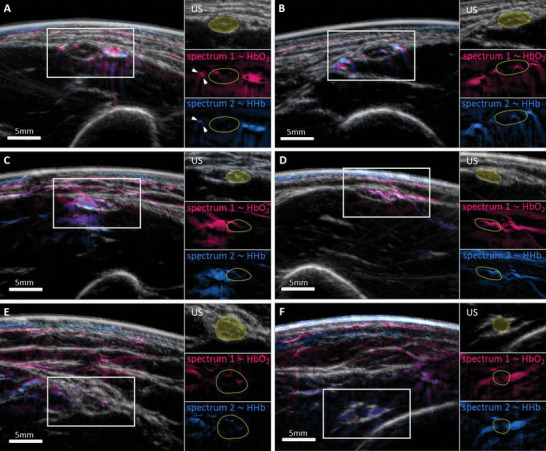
Visualizing the vasa nervorum. Overlay images of ultrasound (US, gray) and two spectral OA components associated with oxygenated (spectrum 1, red) and deoxygenated (spectrum 2, blue) hemoglobin. The single components in the regions outlined with rectangles are shown in three small panels next to the images with the nerve highlighted in yellow. A,B) Two ulnar nerves with intraneural vessels clearly visible in the OA channels. Arteries (presumably the ulnar collateral arteries) and veins at comparable depths can be spectrally distinguished. C,D) Two ulnar nerves with vessels branching into the nerve. E) A median nerve with visible intraneural vessels. F) A radial nerve with two accompanying vessels of medium size, and a small (presumably epineurial) vessel on top.
